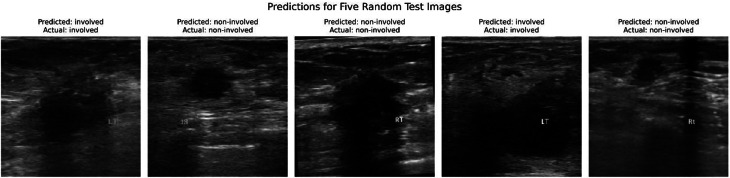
Prediction of the model from ultrasound images
